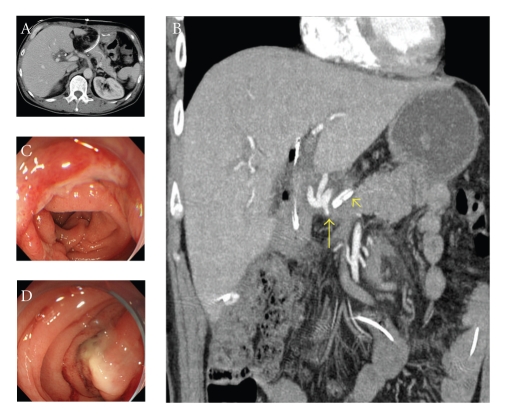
Imaging after sentinel bleeding. (a) CT scan on the 16th day postsurgery shows no abscess or hematoma around the pancreatic anastomosis. (b) The stump of the GDA is 6.0 mm in diameter (arrow), and very close to the drain placed at the anterior aspect of the pancreaticojejunostomy (arrowhead). (c) and (d) an upper endoscopy on the 17th day postsurgery shows ulcerative changes without massive hemorrhage in the gastrojejunostomy (c) and the pancreaticojejunostomy (d).
